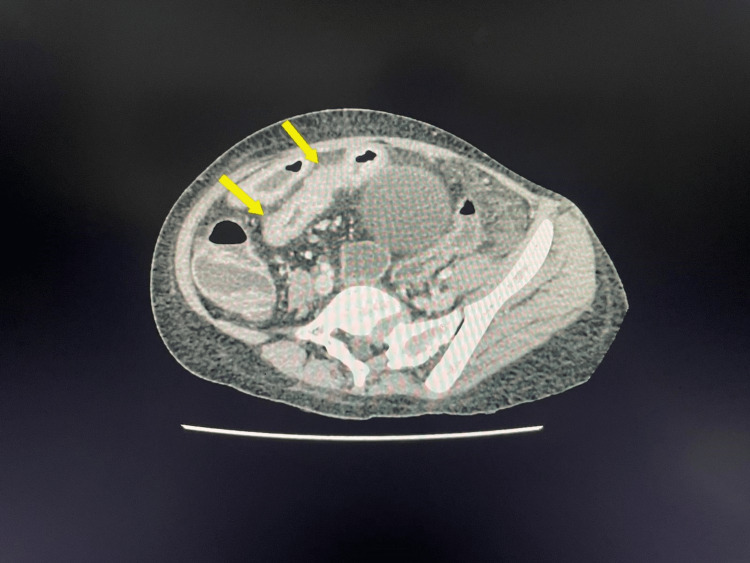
Contrast enhanced computed tomography (CECT) of the abdomen and pelvis The yellow arrows show long and short segments of small bowel wall thickening with abnormal mucosal enhancement (skip lesions) mainly involving the ileal loops.
